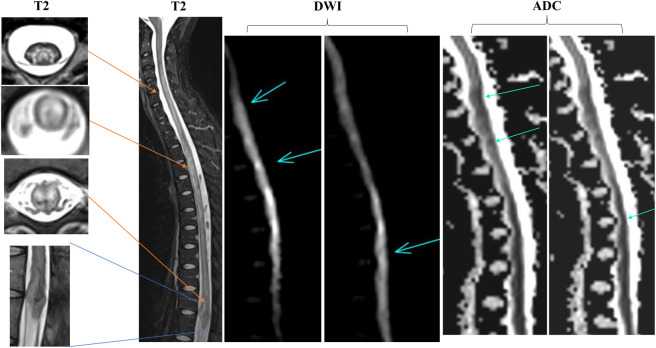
MRI Scans From a Patient With SLE and a Positive Anti-Cardiolipin Antibody With “Double-Seronegative” Myelitis The patient had an acute onset to nadir progressing to paraplegia within hours. DWI/DTI and ADC maps demonstrate focal areas of diffusion restriction (turquoise arrows) suggestive of infarct. T2 MRI sequences show an admixture of cord signal abnormalities that can be seen with both inflammatory myelopathy and spinal cord infarct, including an area of T2 hypointensity within the lumbar cord/conus suggestive of hemorrhage (blue lines; lower). No source for thromboembolism or primary vascular anomaly was found on the spinal MR angiogram. Serum AQP4-IgG and MOG-IgG were negative by live CBA. The CSF profile was inflammatory (WBC 65 cells/mm^3^, protein 66 mg/dL) with no oligoclonal bands, and there was poor response to acute immunomodulatory therapy with a mRS score of 4 at follow-up. ADC = apparent diffusion coefficient; AQP4 = aquaporin-4; CBA = cell-based assay; DTI = diffusion tensor imaging; DWI = diffusion-weighted imaging; MOG = myelin oligodendrocyte glycoprotein; mRS = modified Rankin Scale; SLE = systemic lupus erythematosus; WBC = white blood cell.
